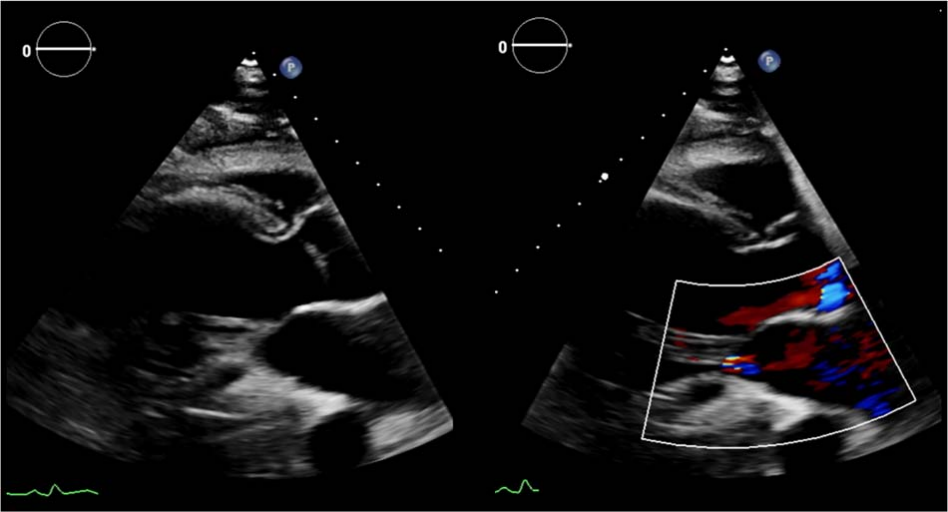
Preoperative echocardiography: large LV aneurysm below the posterior mitral valve leaflet tethering it open in systole resulting in severe functional mitral regurgitation.
